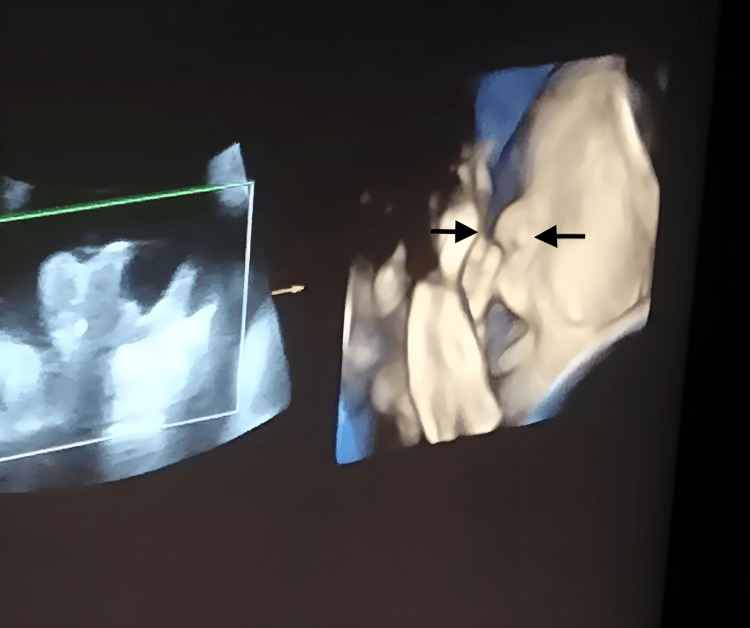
4D Ultrasound view of twin II at 31 weeks of gestation demonstrating absence of the nasal bone. The 4D ultrasound shows the facial anatomy of twin II at 31 weeks of gestation. The nasal bone is not visualized in the expected region, consistent with its absence [7].
